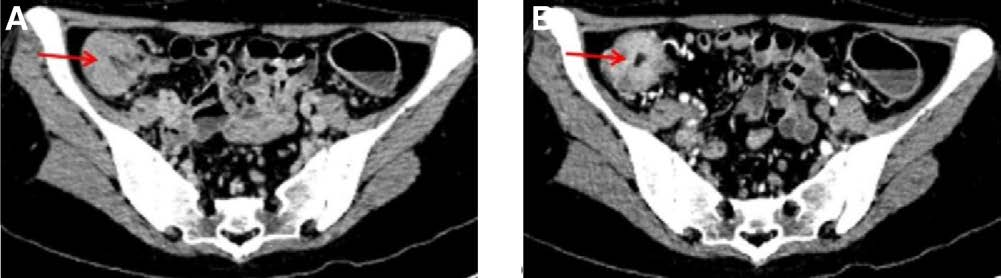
Abdominal enhanced CT images. The images show thickening of the (A) ileocecal and (B) colorectal walls (arrows). CT = computed tomography.
